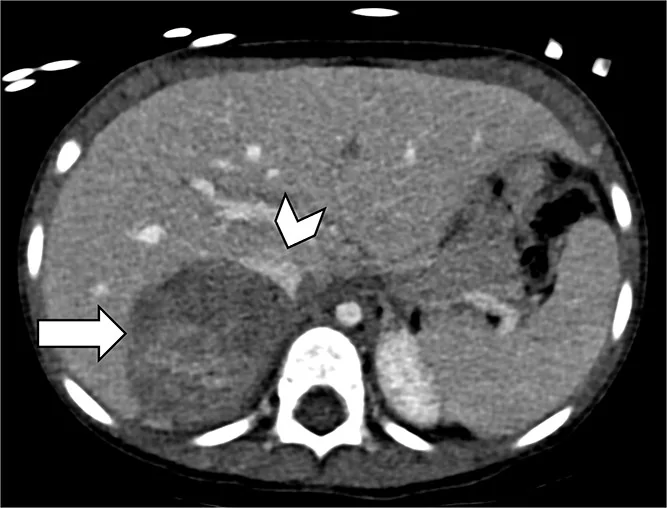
Axial CT image of the abdomen shows a 5 cm heterogenous right adrenal mass (arrow) with narrowing of the IVC that remains patent (arrowhead).
